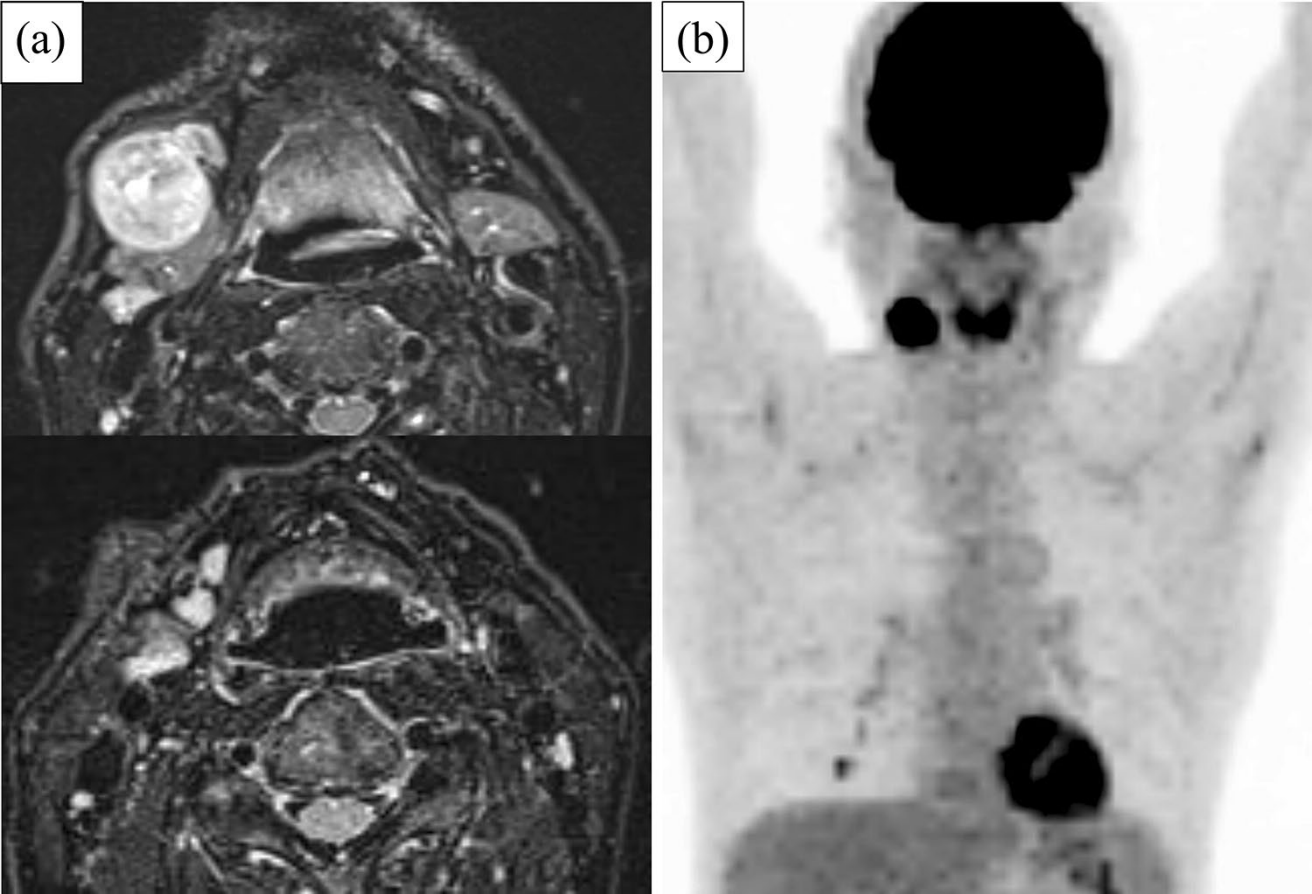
Magnetic resonance imaging and maximum intensity projection positron emission tomography (PET) images at initial diagnosis. a Short TI inversion recovery images showed a lymph node (LN) of approximately 2.7 cm in maximum diameter in the right submandibular region and LNs of approximately 1.0 cm in the right upper jugular region. b PET showed significant positive fluorine-18-2-deoxy-D-glucose accumulation in the LNs in the right submandibular and upper jugular regions
